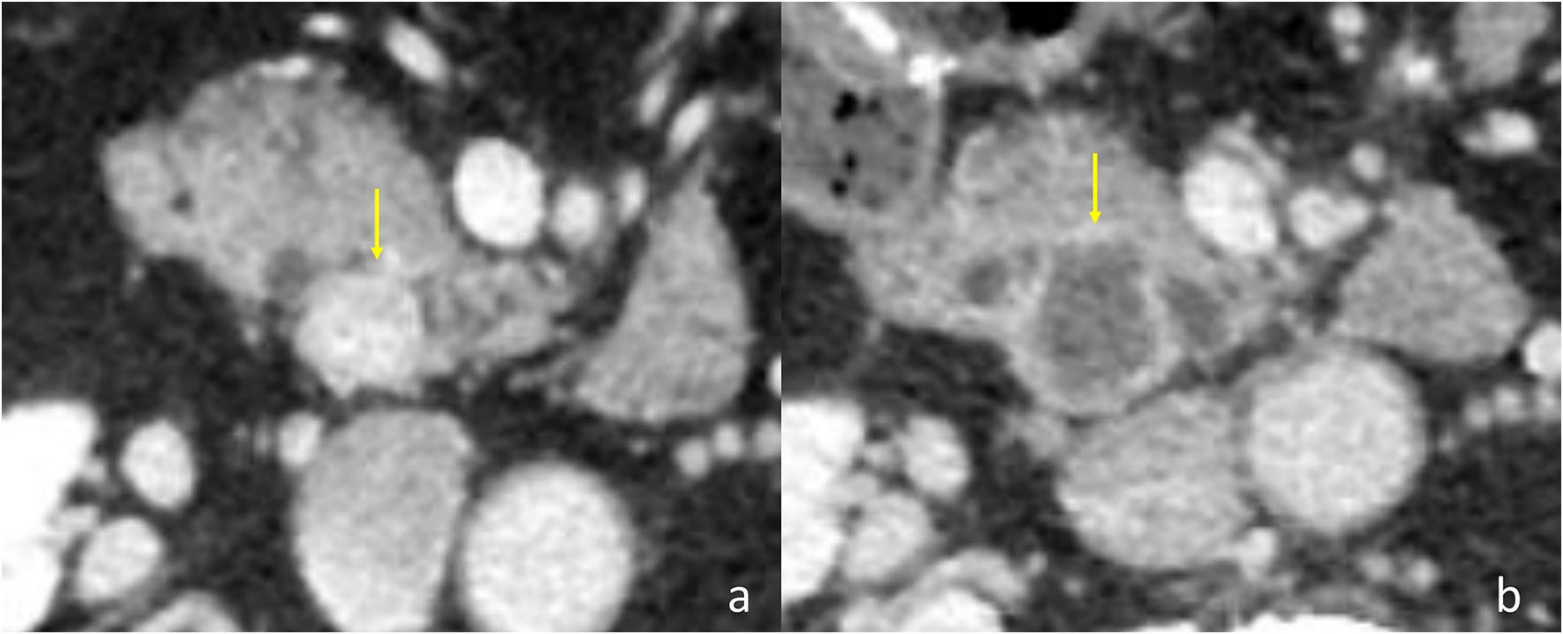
a: Contrast computed tomography (CT) imaging showed a 12-mm-sized plethoric tumor in the uncus of the pancreas (arrow). b: On admission, Contrast CT showed a 22 mm-sized ring-shaped enhancement tumor in the uncus of the pancreas (arrow)
